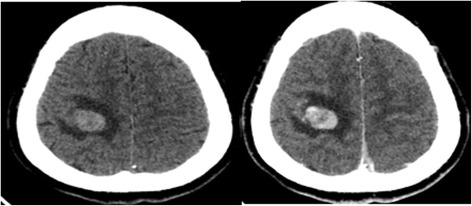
The brain CT scan shows multiple round nodules with peripheral enhancement. The largest is in the right parietal lobe and measures 1.5 cm × 1.9 cm
